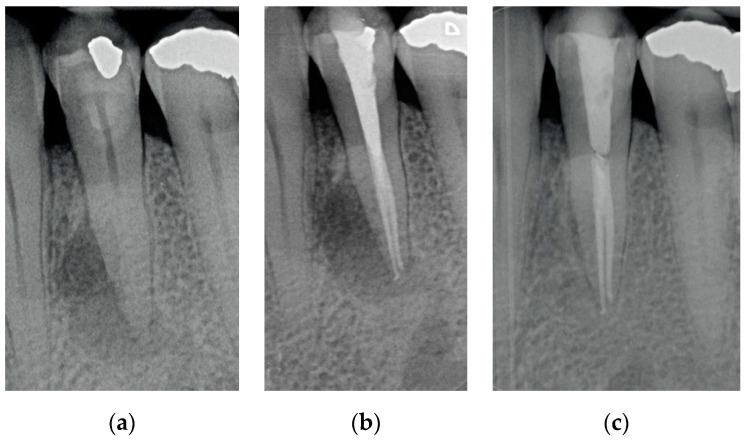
(a) A lower bicuspid in need to be endodontically treated because of necrosis of the pulp and periapical lesion; (b) The tooth after being endodontically treated. Two canals were found, cleaned and filled; (c) The tooth at 2 years recall. The periapical lesion completely disappeared.
